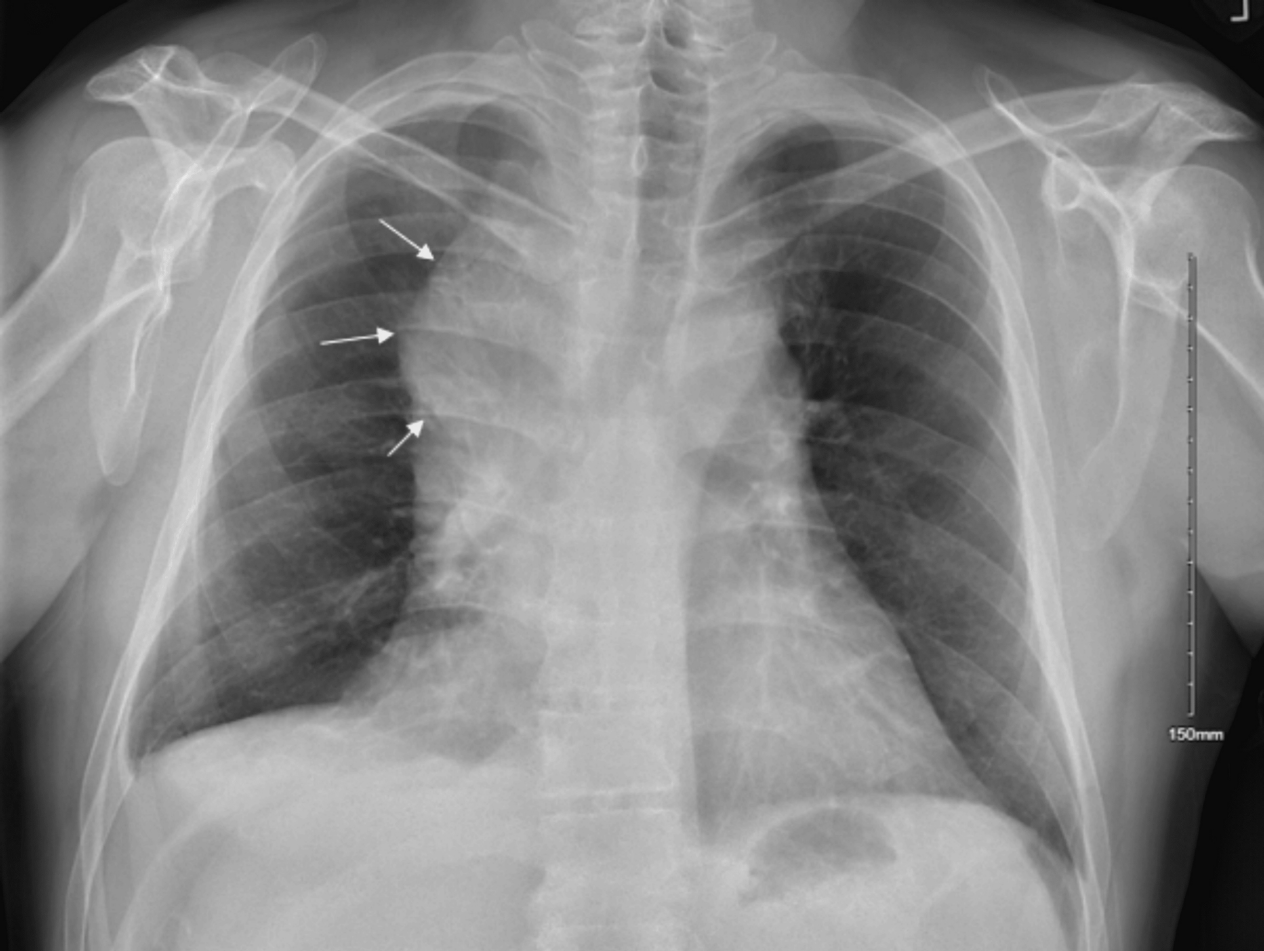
Chest radiograph of the patient showing right-sided suprahilar mass-like lesion.
